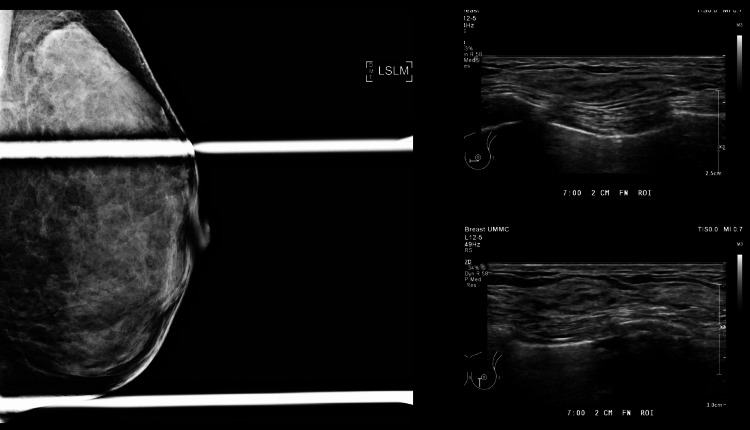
Post eight cycles of chemotherapy reassessment study showing non-visualisation of the left breast primary lesion and tumour marker on both ultrasound and mammogram.
